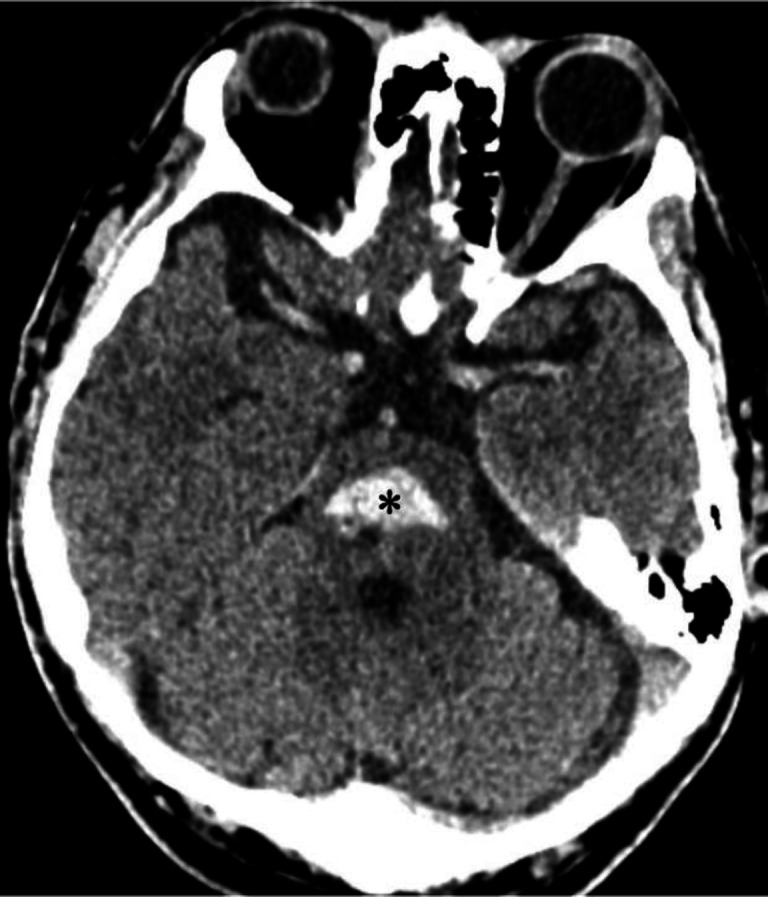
Non-contrast CT brain of a 76 year-old male with uncontrolled hypertension who was unconscious when brough to hospital after developing an acute headache. The image shows a hyperdense region centrally at the pons (asterisk) suggestive of a hypertensive haemorrhage
